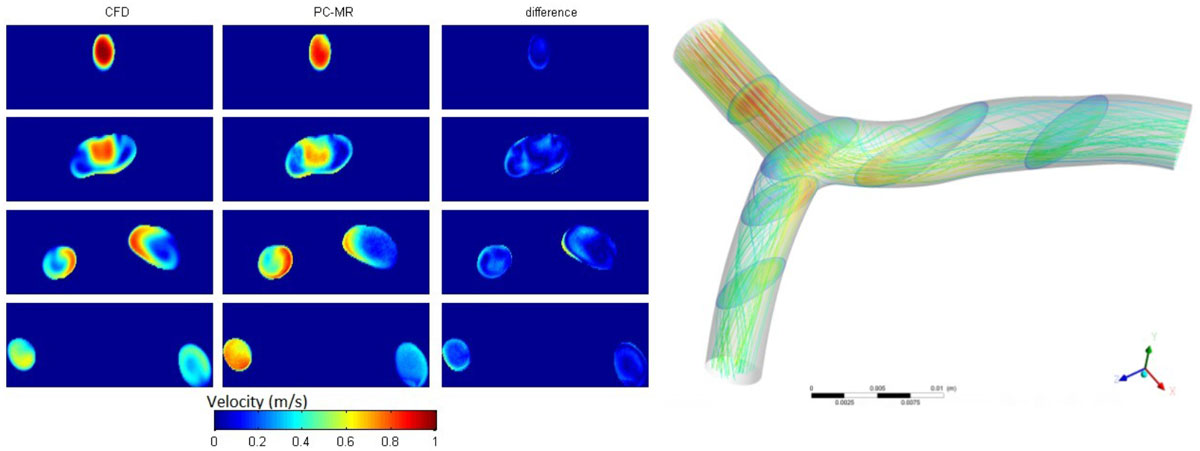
Detailed flow comparison for the 110 degree patient bifurcation with i) velocity norm contours of real-scale CFD (left), dynamically scalled PC-MRI (middle) and their difference (right) in ii) four planes of the flow field volume.
